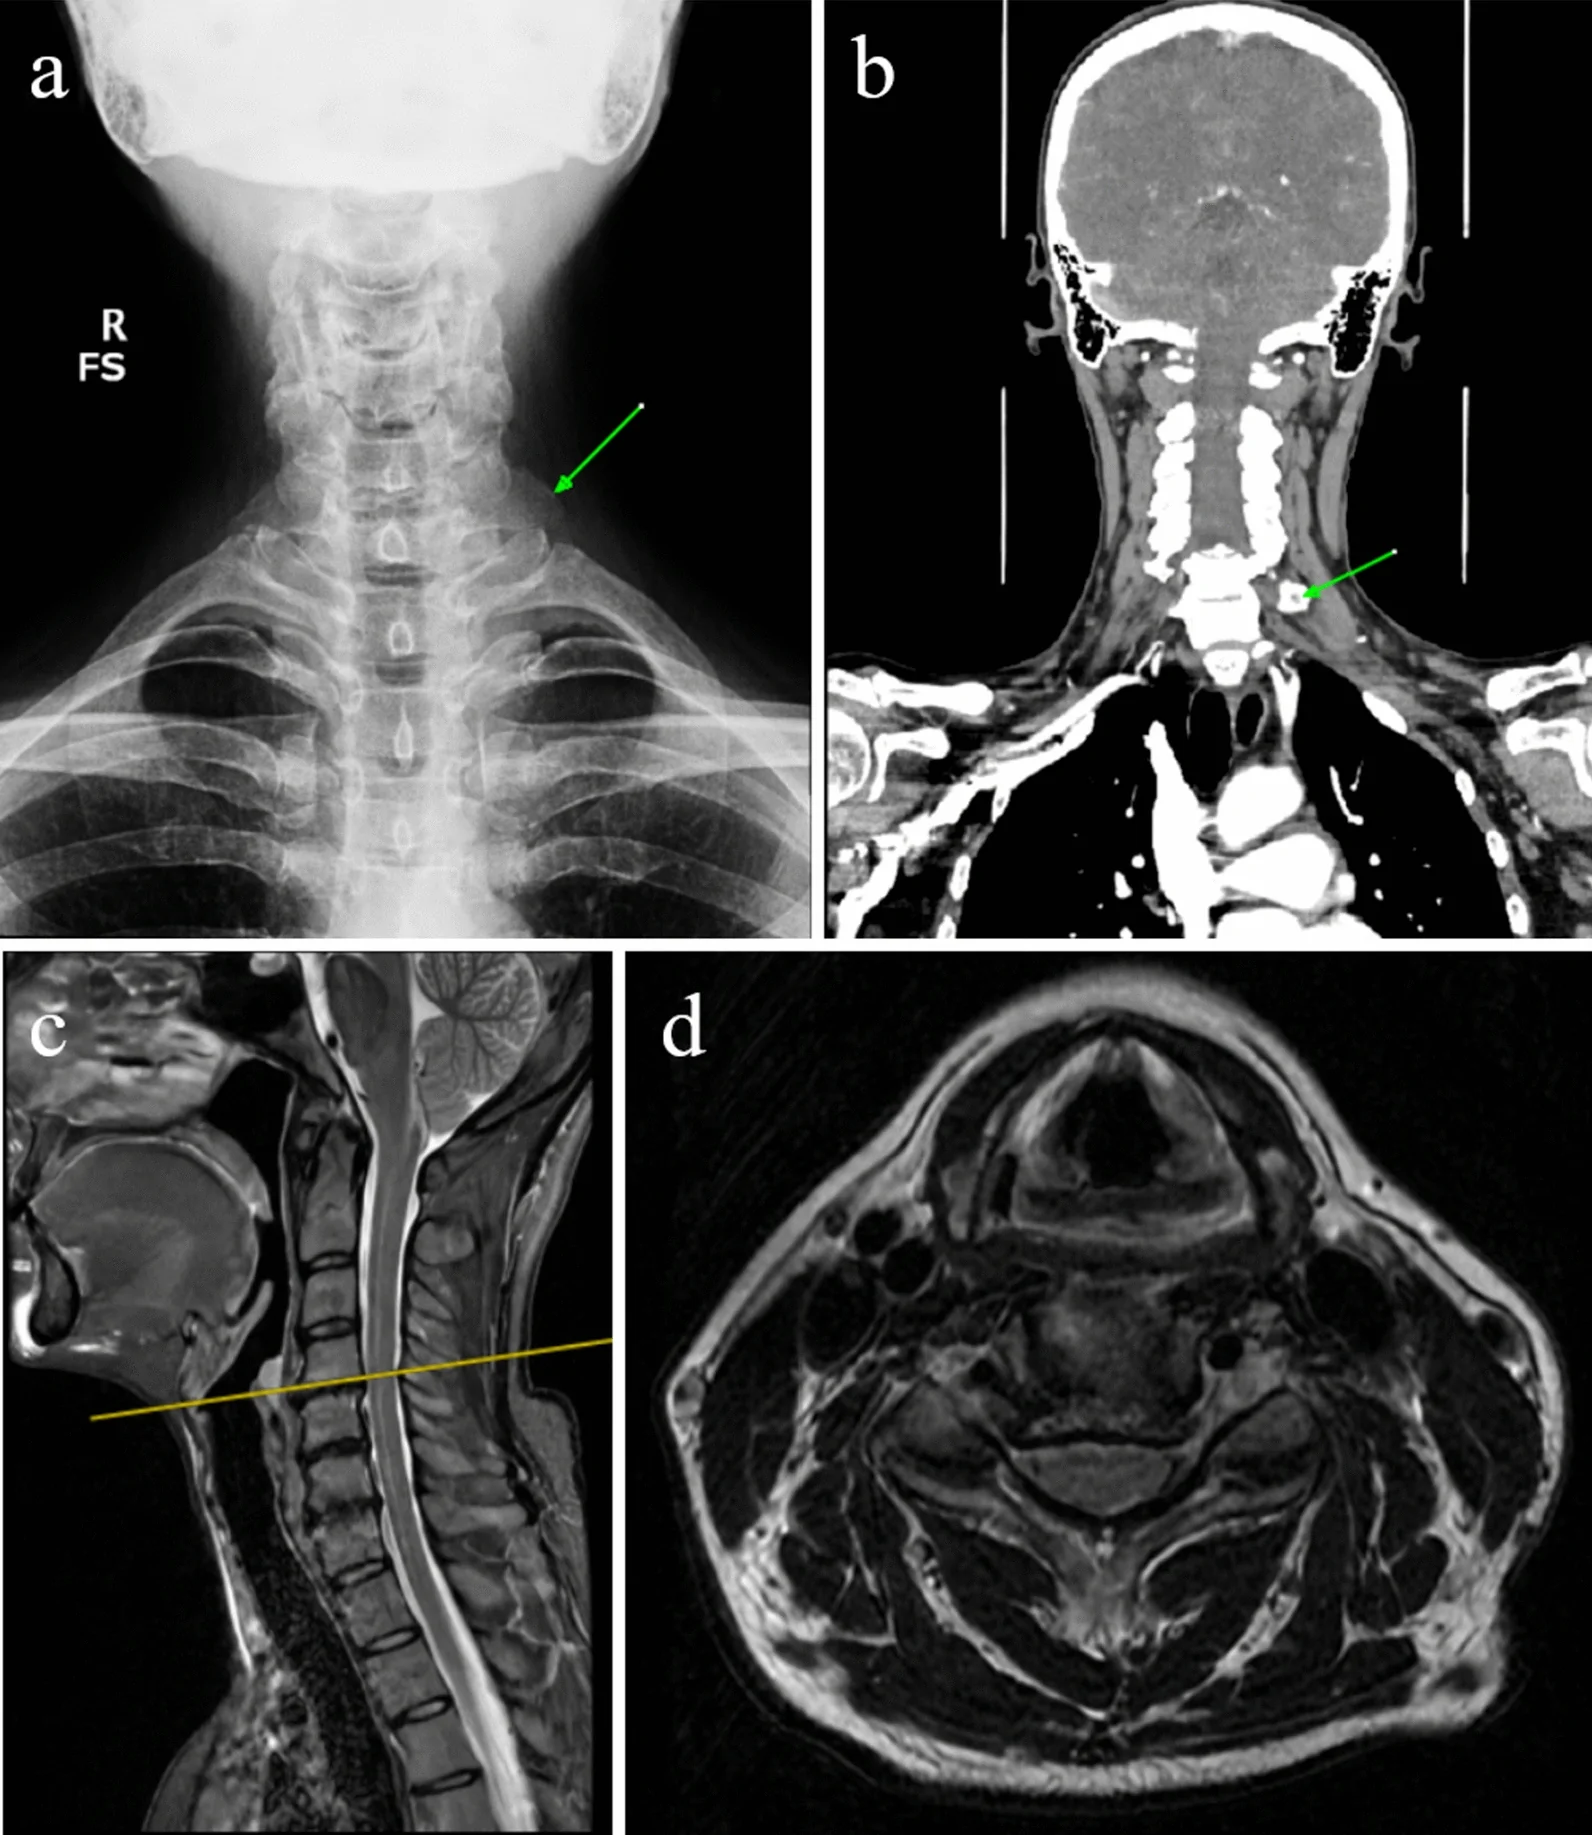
Anteroposterior cervical X-ray acquired following the initial presentation (a) and coronal reconstruction of a cervical computed tomography arteriogram acquired following the therapeutic injections (b) showing elongated and hypertrophic left transverse process of C7 (arrow). The process is seen violating the left interscalene space and contacting neuromuscular structures. c, d Sagittal T2 FSE STIR (c) and corresponding Axial T2 FSE (d) sections showing severe C4–C5 foraminal narrowing on the right side and a mild narrowing on the left side. The patient’s symptomatology is on the left side
